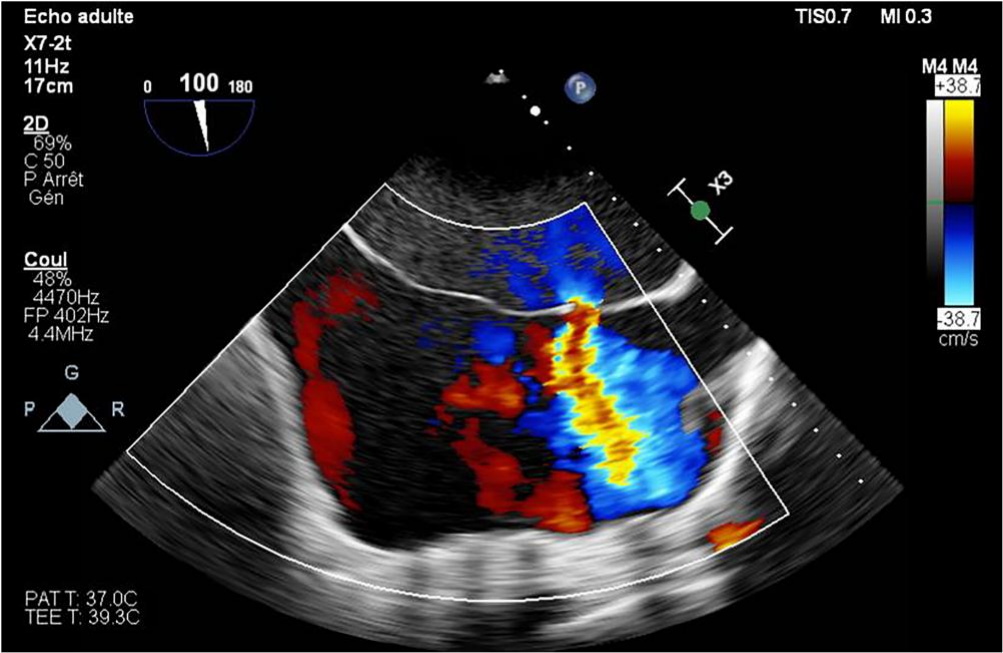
Transoesophageal echocardiogram bicaval view showing the shunt flow through the ostium secundum atrial septal defect.
